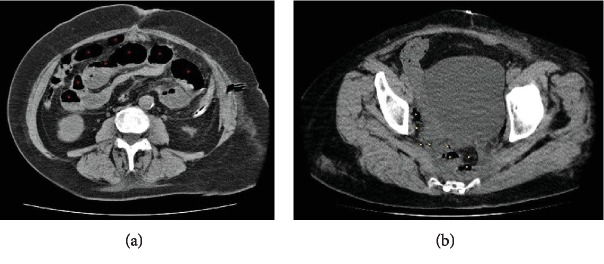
Patient's CT scans on the 6^th^ postoperative day. Multiple intestinal “levels” of gas and fluid throughout the abdominal cavity were observed ((a), red arrowheads). Part of the distal intestinal loop with difference in lumen diameters was situated at the level of the pelvic floor ((b), yellow arrowheads) and was adherent to both rectal and vaginal stumps.
